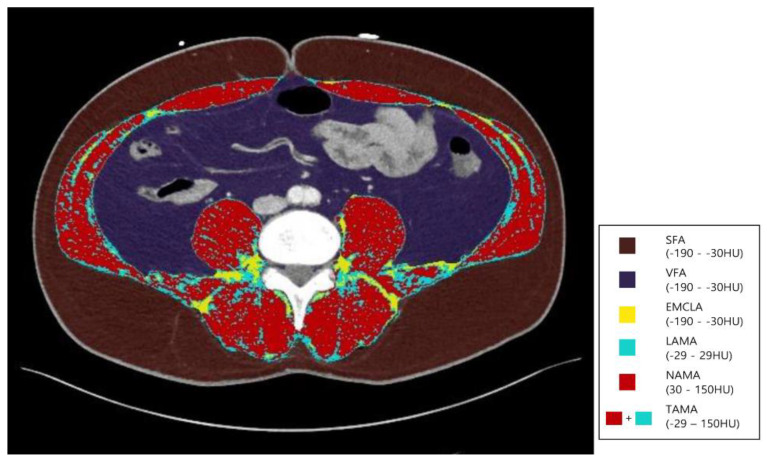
Segmental abdominal body fat and muscle analysis at the L3 vertebra on abdominopelvic computed tomography. HU, Hounsfield units; IMAT, intermuscular adipose tissue; LAMA, low-attenuation abdominal muscle area; NAMA, normal-attenuation muscle area; SFA, subcutaneous fat area; TAMA, total abdominal muscle area; VFA, visceral fat area.
